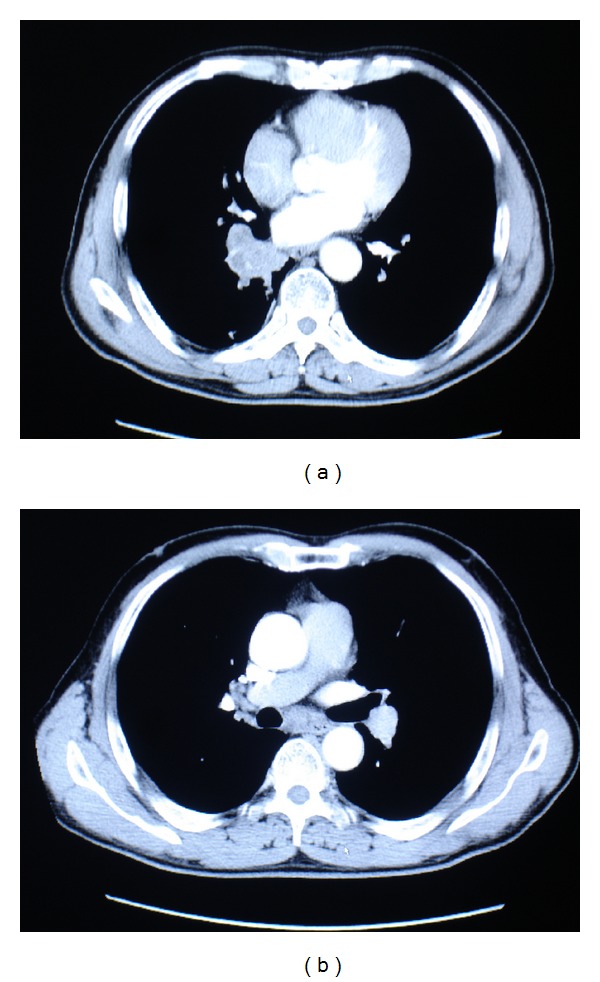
Preoperative chest CT scan revealed a mass in the right lower lobe (a), with enlargement of mediastinal lymph nodes (b).
